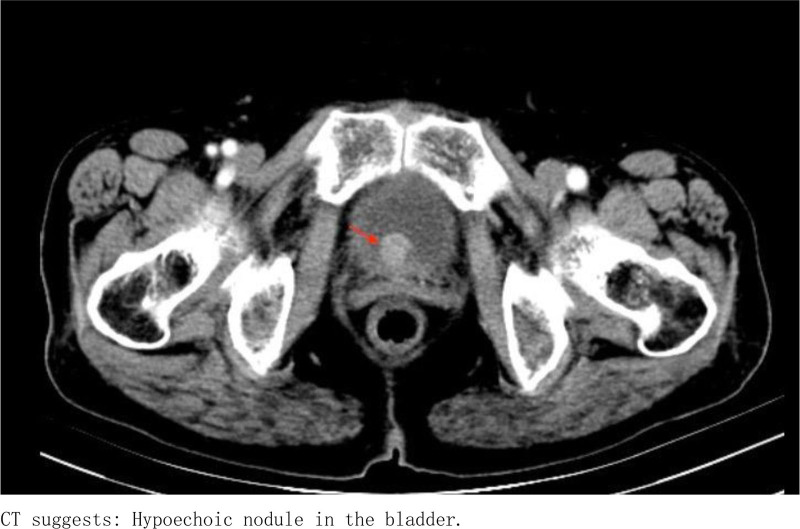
CT suggests: hypoechoic nodule in the bladder. CT = computed tomography.
